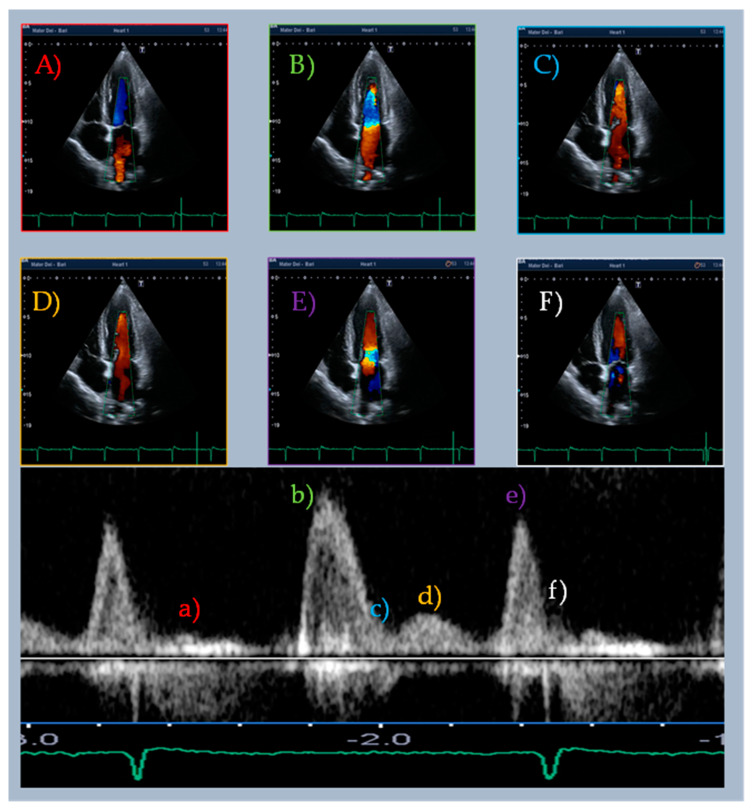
Color Doppler imaging for the analysis of the L wave and flow pathway. The upper panels (A–F) show the transmitral and right upper pulmonary vein flow sampled simultaneously in the color Doppler apical four-chamber view. The lower panel shows the corresponding transmitral flow sampled in pulsed-wave Doppler (a–f). (A) (a) Systolic phase: anterograde pulmonary vein flow and mitral valve closure. (B,C) Early diastolic phase: anterograde mitral and pulmonary vein flows, (b,c) E wave. (D) Diastasis: transmitral mid-diastolic flow and continuous pulmonary vein flow, (d) L wave. (E) End-diastolic phase: increased mitral flow velocity and no pulmonary vein flow, (e) A wave. (F) Early systolic phase: mitral valve closure.
